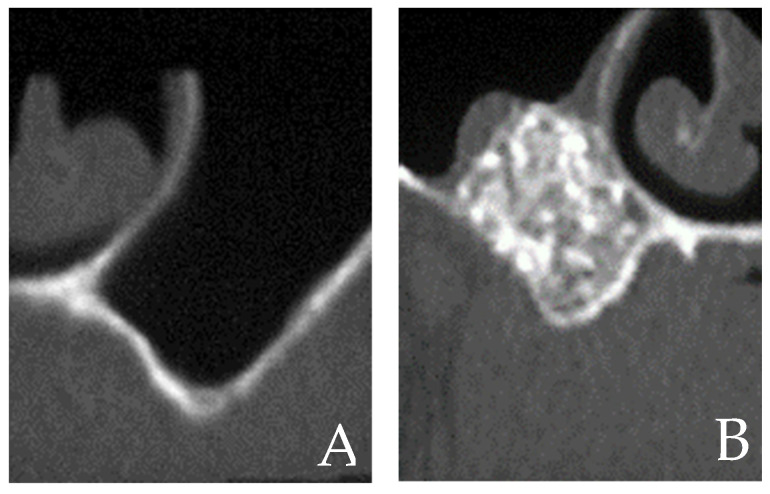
Section of a CBCT before (A) and after (B) the sinus lift.
